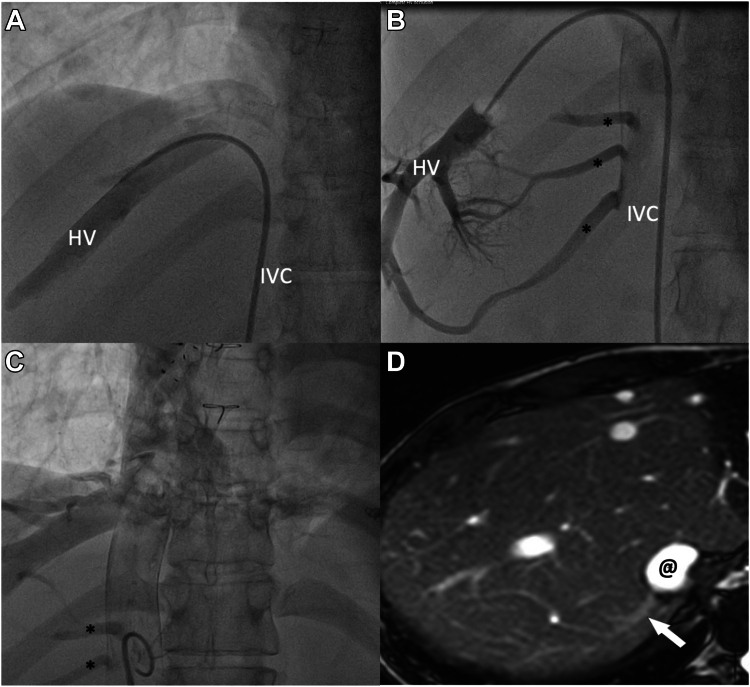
Hepatic venovenous collaterals. (A) Nonocclusive hepatic venous angiography, (B) occlusive hepatic venous angiography demonstrating bridging collaterals or accessary hepatic veins (∗) con necting the inferior vena cava, (C) inferior vena cava angiogram demonstrating these accessary hepatic veins, and (D) transverse b-SSFP MR image demonstrating one of the accessory hepatic veins (arrow) draining into the inferior vena cava (@) in segment VII. b-SSFP MR, balanced steady-state free precession magnetic resonance; HV, hepatic vein; IVC, inferior vena cava.
